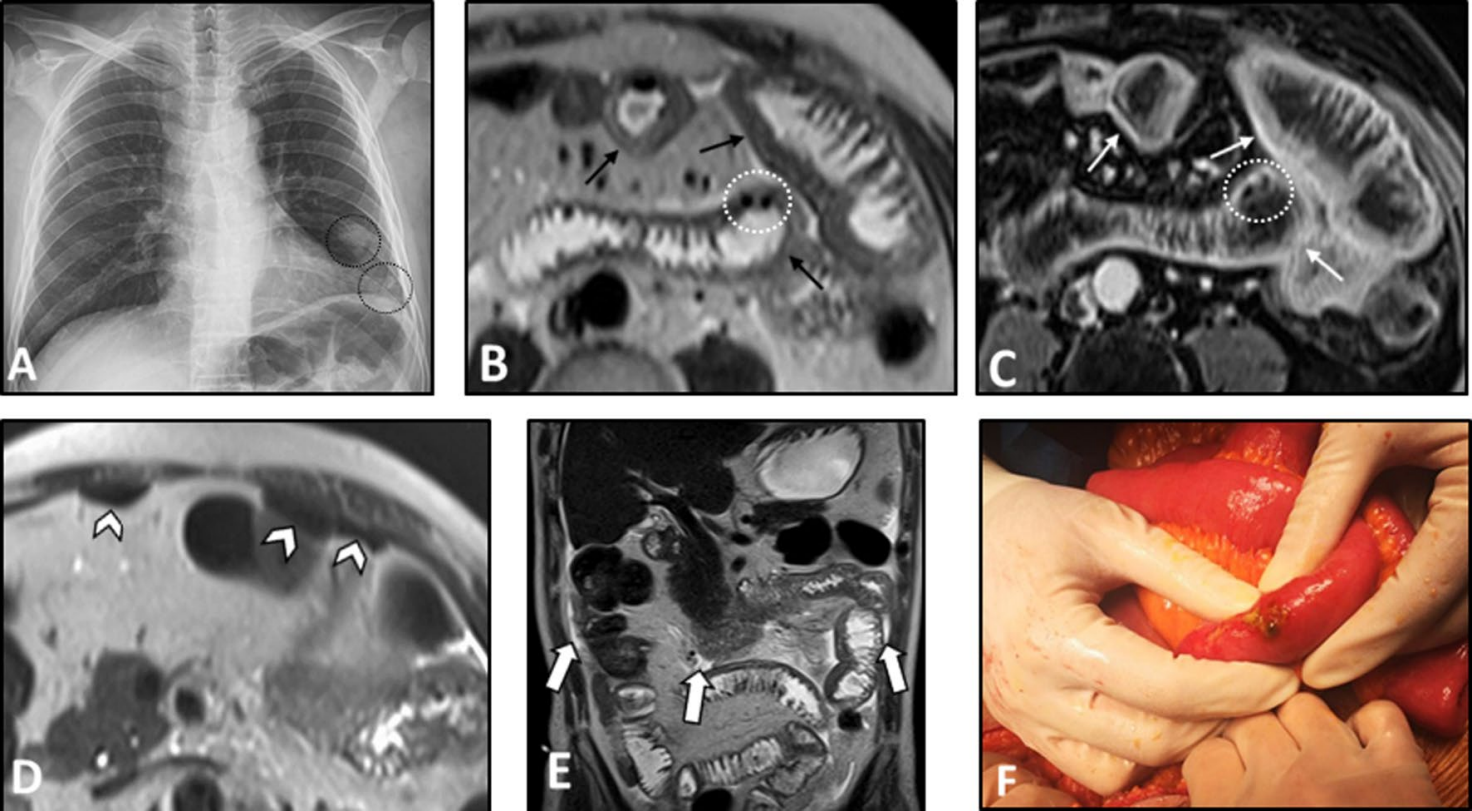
Intestinal vasculitis and perforation in a 56-year-old male with known ANCA-positive granulomatosis with polyangiitis and long history of immunosuppressive therapy presenting with acute abdominal pain and leukocytosis. Chest X-ray (A) shows two pulmonary nodules projecting over the lower zone of the left hemithorax (black dotted ovals). MRE was obtained. Axial T2-W image (B) reveals multi-focal segmental mural thickening in jejunal loops (thin black arrows). Axial post-contrast T1-W image (C) displays intense mural hyperenhancement (thin white arrows) in the involved segments. Focal intestinal pneumatosis (white dotted ovals) is also evident. Axial T2-W image (D) shows pneumoperitoneum (white arrowheads) at the non-dependent portion of the abdominal cavity, indicating bowel perforation. Coronal T2-W image (E) demonstrates small amount of free fluid (thick white arrows) at the abdominal cavity. Due to acute nature of presentation, the patient subsequently underwent laparotomy. Intraoperative photograph (F) reveals focal bowel necrosis and perforation. No signs of peritonitis were found in the initial examination, more likely due to the immunocompromised status of the patient
